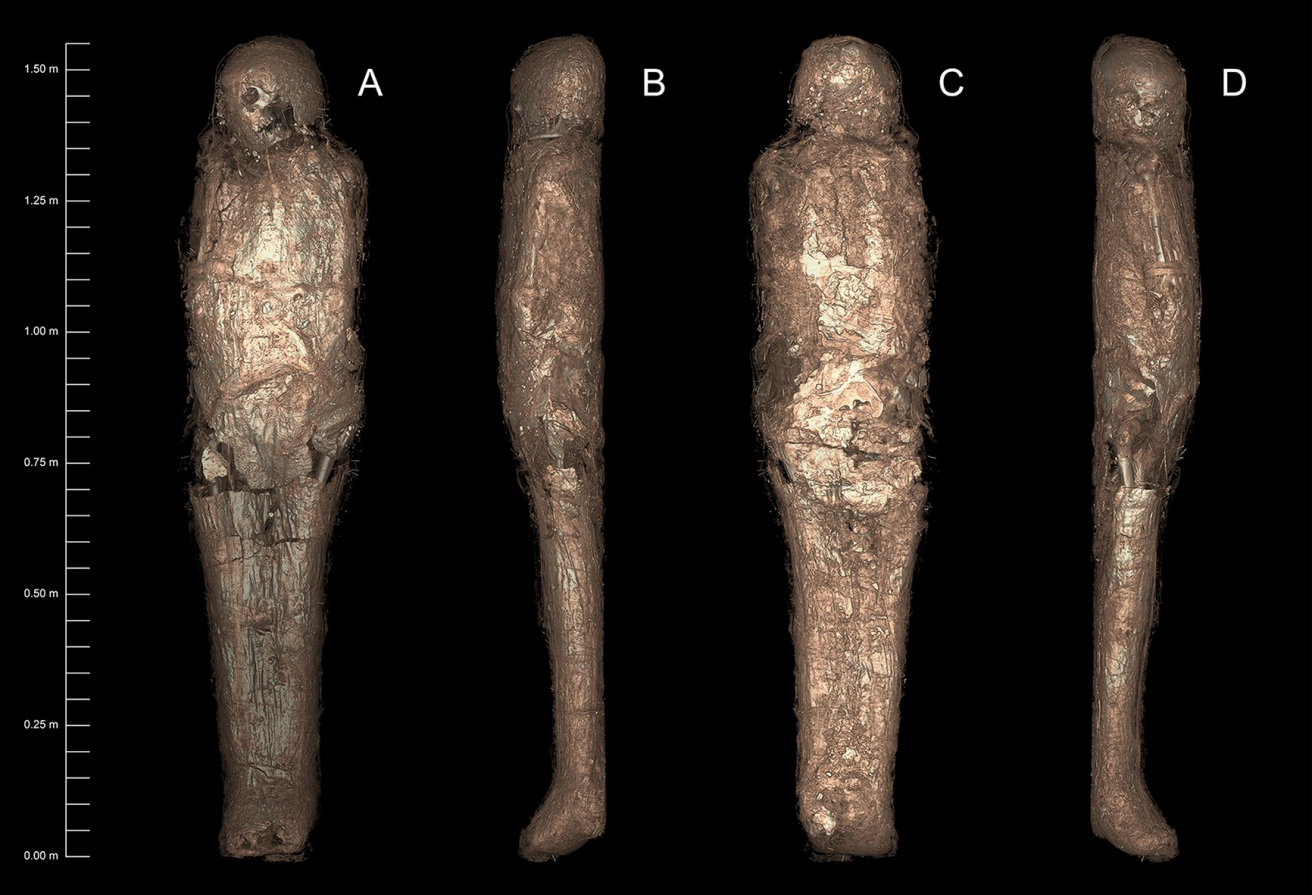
3D-rendered CT images of mummified individual (NMR.27.3) showing the carapace and broken sections (stitched image). A. Anterior. B. Lateral left-hand side. C. Posterior. D. Lateral right-hand side. (courtesy Chau Chak Wing Museum and Macquarie Medical Imaging).
